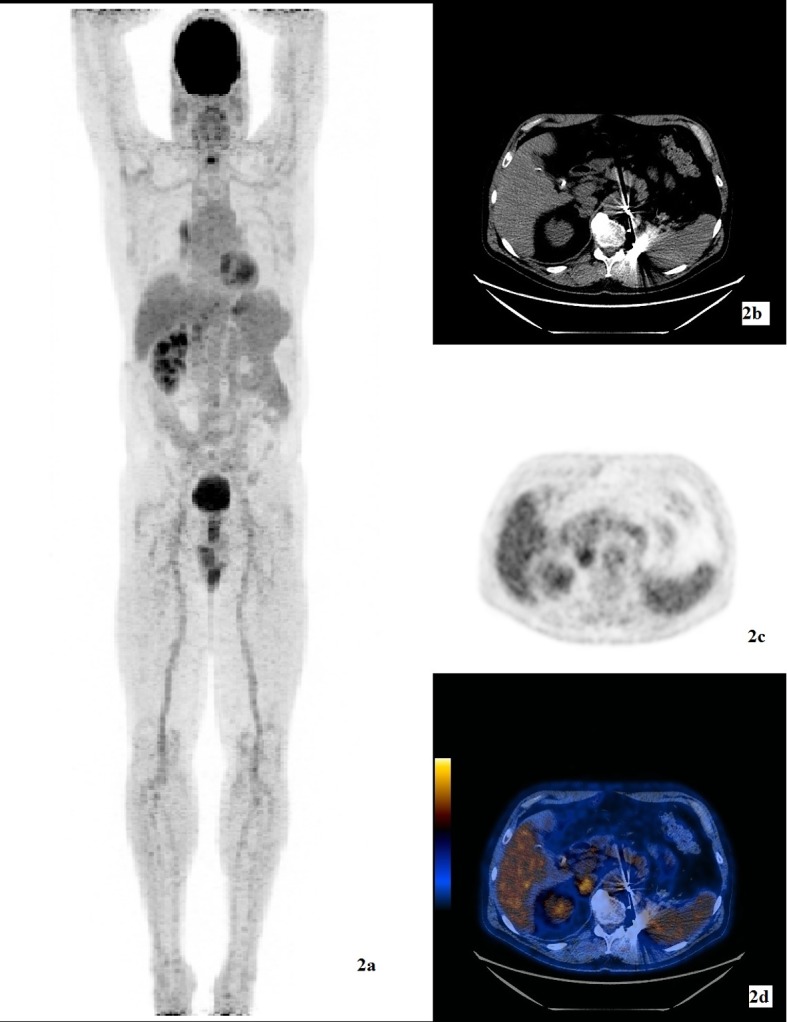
(a) 3D MIP image of FDG-PET/CT study after the laparoscopic cholecystectomy. (b) CT transmission image for attenuation correction and anatomic localization. (c) FDG emission image. (d) Hybrid FDG-PET/CT image showing inflammatory changes after laparoscopic cholecystectomy but no signs of local recurrence.
